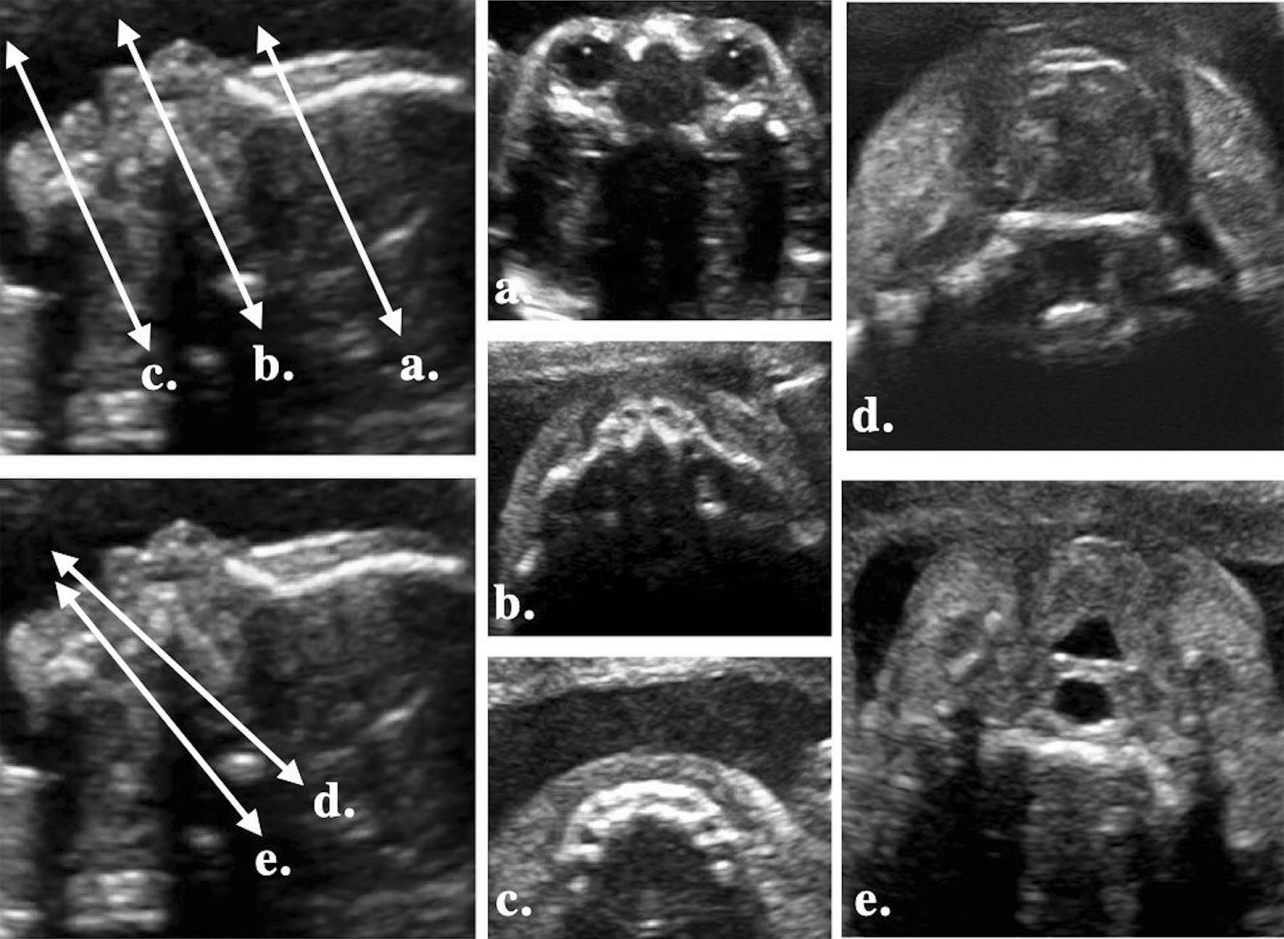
Ultrasound stepped views. To confirm the OFC diagnosis, 5 planes were obtained. (a) Eye sockets (b) upper alveolus (c) lower alveolus (d) posterior edge of hard palate (f) inferior part of soft palate.
